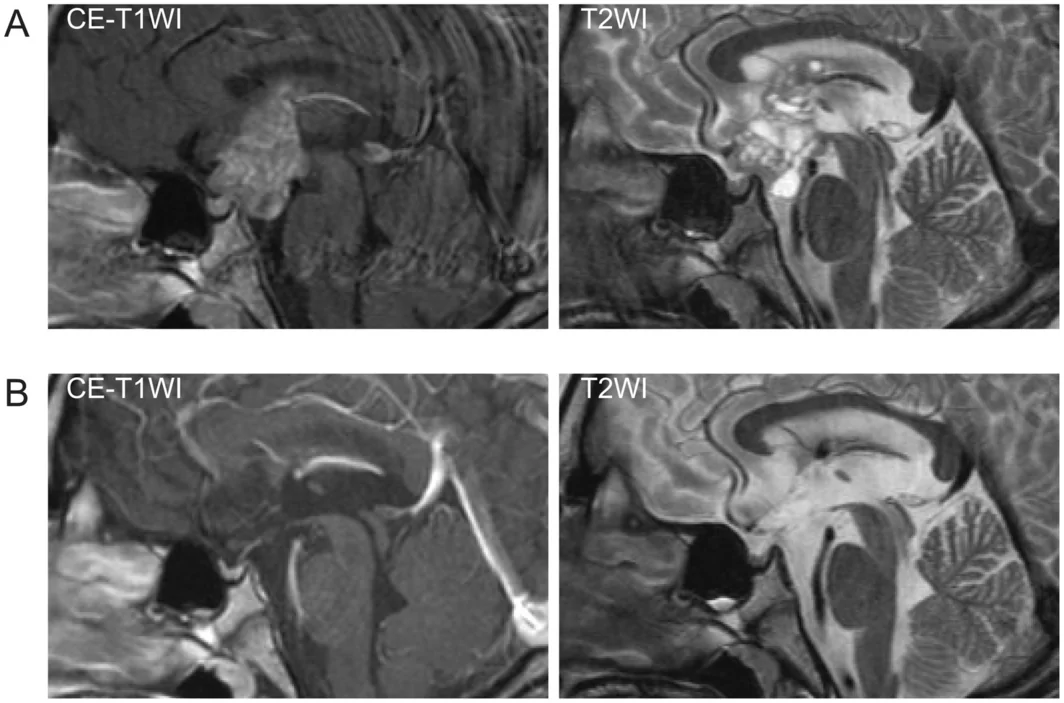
Brain magnetic resonance imaging (MRI) before and after treatment. (A) Pre‐treatment contrast‐enhanced T1‐ and T2‐weighted MRI demonstrating a suprasellar mass extending toward the third ventricular and pineal regions, involving the hypothalamic region. (B) Post‐treatment contrast‐enhanced T1‐ and T2‐weighted MRI after completion of chemoradiotherapy showing marked tumor regression.
